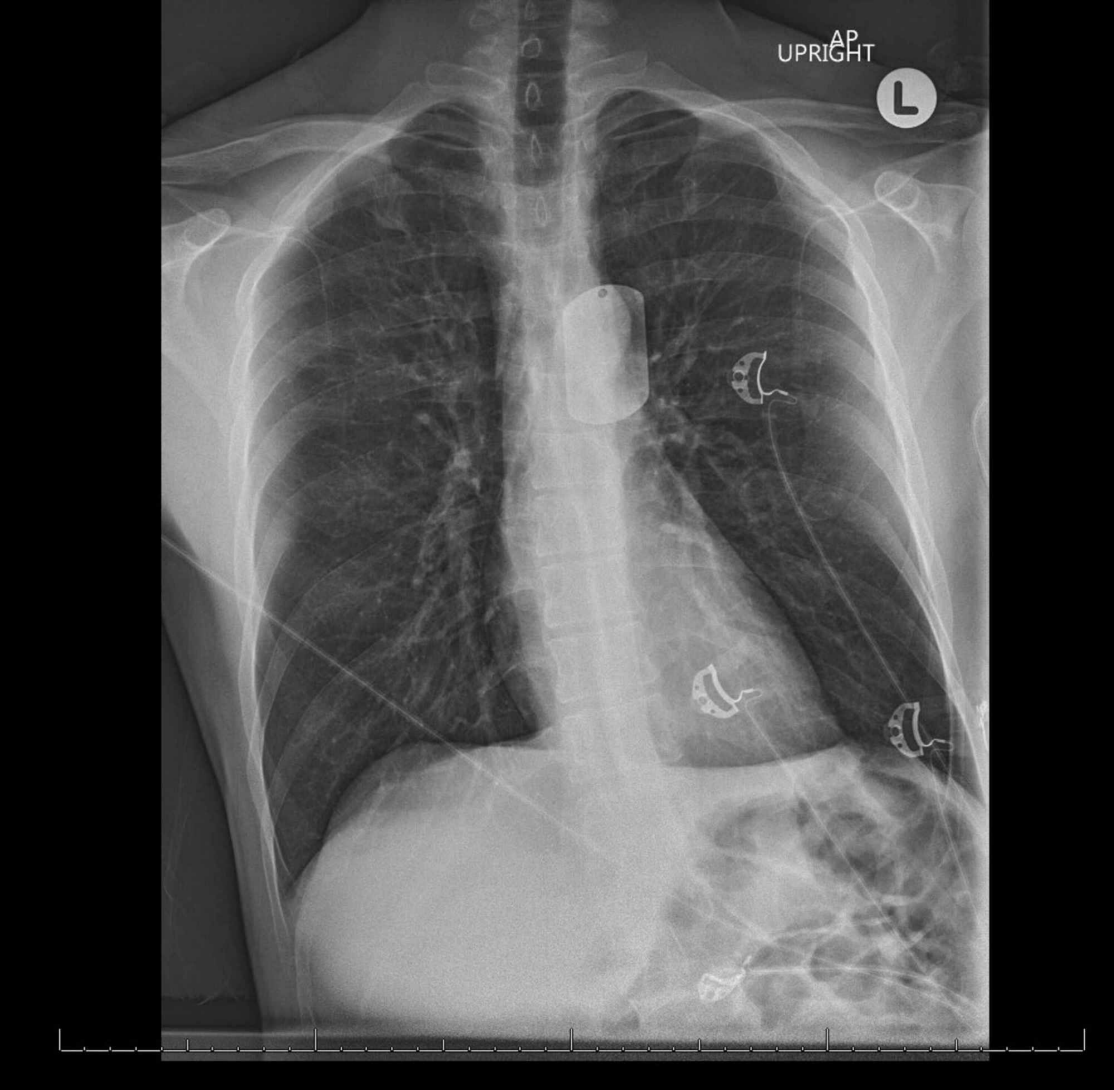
An unremarkable anteroposterior chest radiograph on admission
